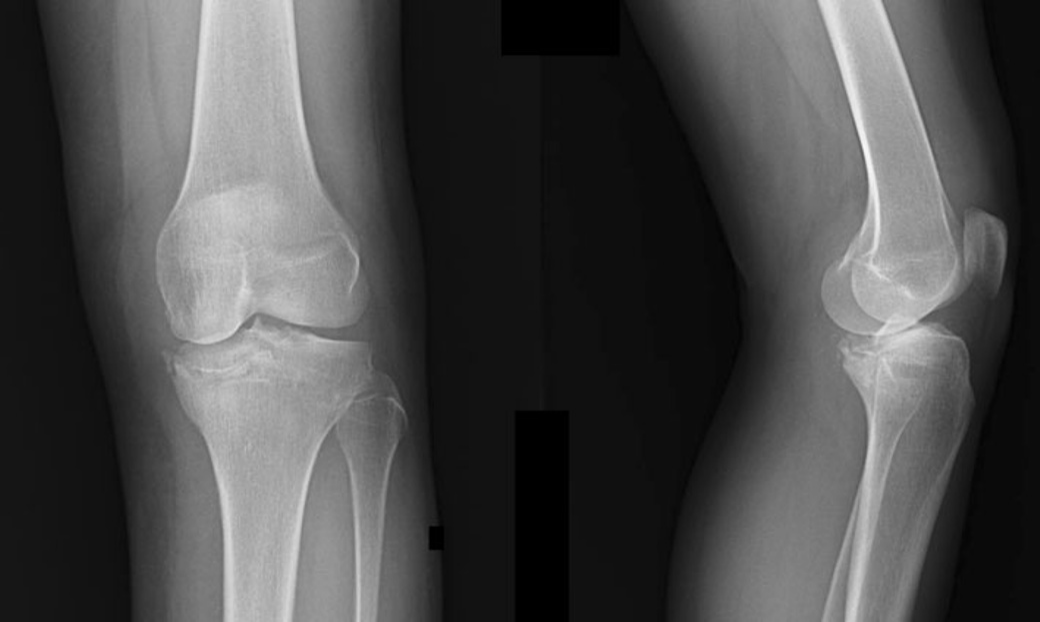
Preoperative X-rays of the left knee showing a Schatzker type IV fracture of the proximal tibia
